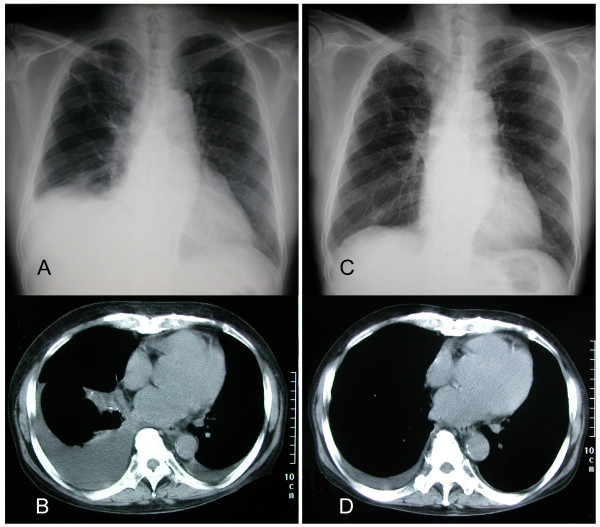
Pleural effusion was significantly alleviated by vincristine, adriamycin and dexamethasone chemotherapy. Chest radiograph (A) and computed tomography imaging (B) showing pleural effusion at admission. Chest radiograph (C) and computed tomography imaging (D) showing significant improvement 30 days after VAD.
